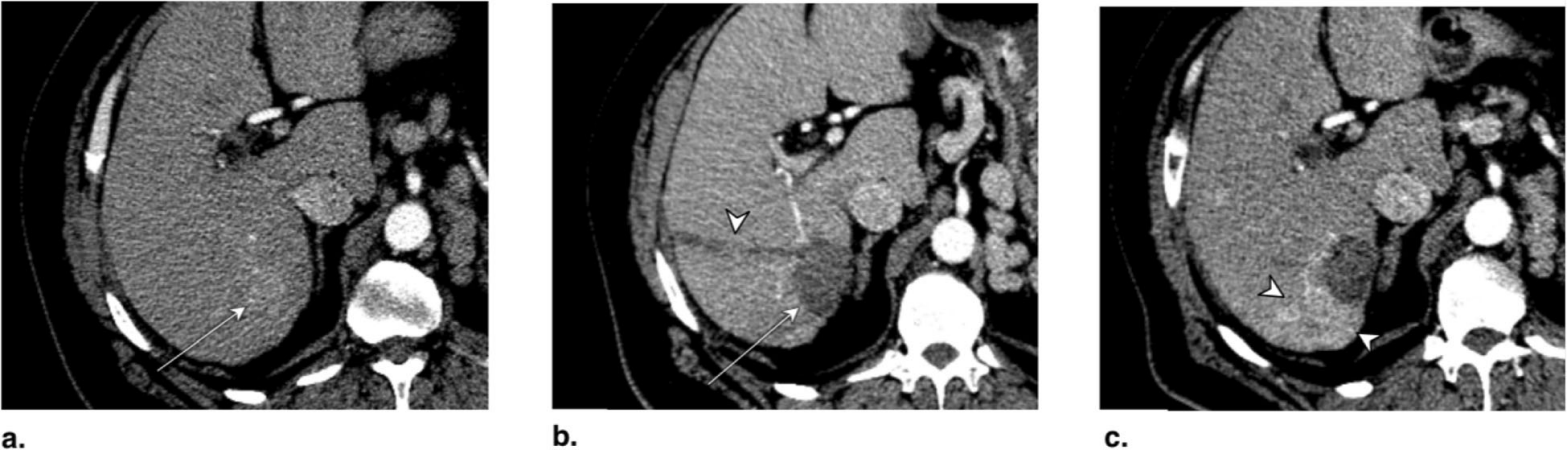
Axial CT in late arterial phase obtained (a) pretreatment: image shows nonrim arterial phase hyperenhancement (APHE) of a right lobe LR-4 observation (arrow). b 2 months post radiofrequency ablation (RFA): image shows needle trajectory (arrowhead) and a treatment cavity (arrow) with no residual tumor. c 8 months post RFA: image shows irregular masslike APHE (arrowheads) posterior to the treatment zone. The treated observation is categorized LR-TR Viable
